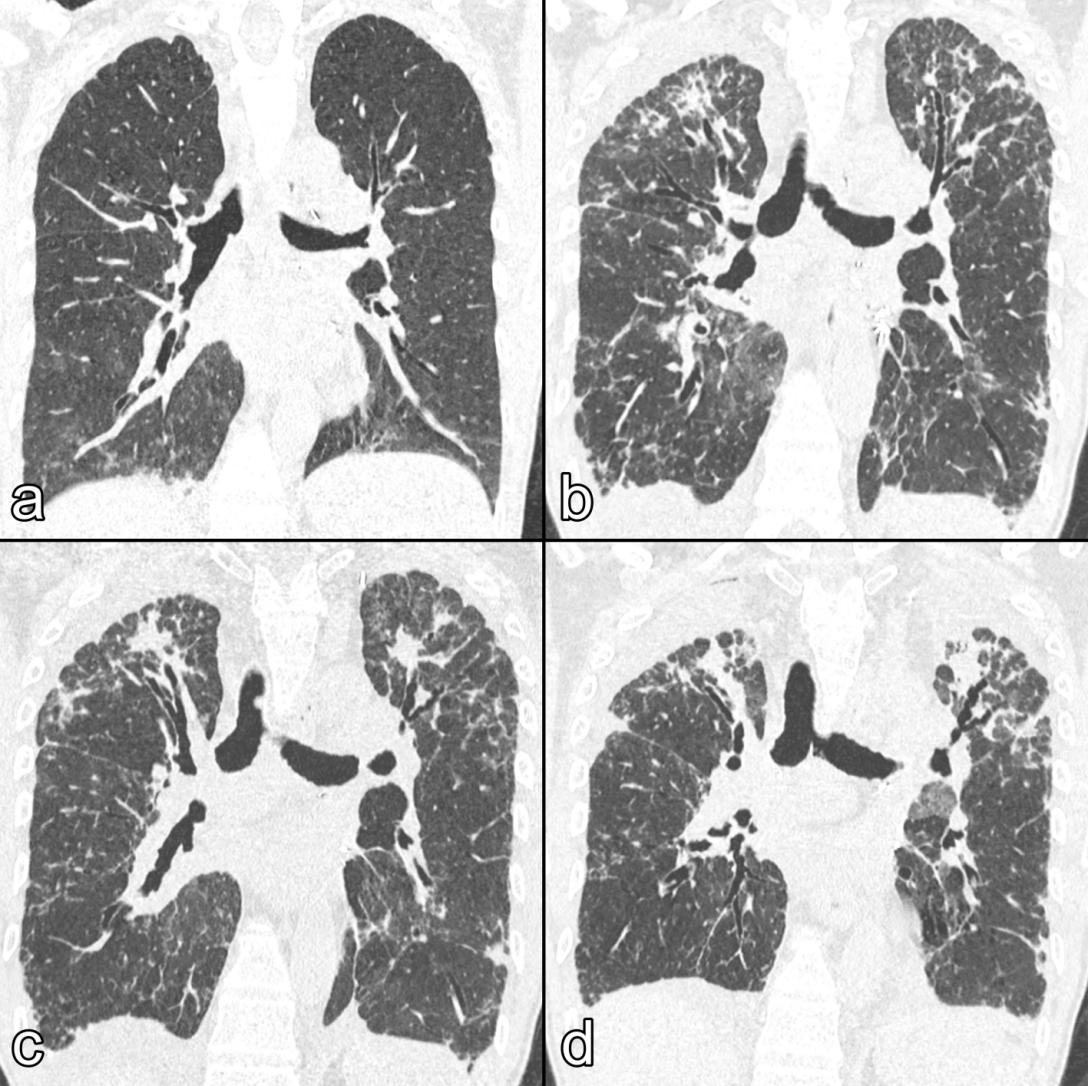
Coronal HRCT images showing the progression of the PPFE relapse after the first bilateral lung transplantation. (a) The first post-operative HRCT shows regular outcome of the transplantation and no signs of bronchial obliterative syndrome. (b) After 4 months, HRCT shows bilateral diffused parenchymal consolidations, with prevalence in the upper lobes and in the subpleural regions, together with some reticular opacities, traction bronchiectasis and mild pleural thickening. (C) 1 month later, HRCT shows increase in both the parenchymal consolidations and pleural thickening, together with mild upper-lobe volume loss. (D) After 3 months, HRCT shows marked pleural thickening together with traction bronchiectasis and severe lung volume loss; at this stage, the patient underwent the second lung transplantation. HRCT, high-resolution CT; PPFE, pleuroparenchymal fibroelastosis.
